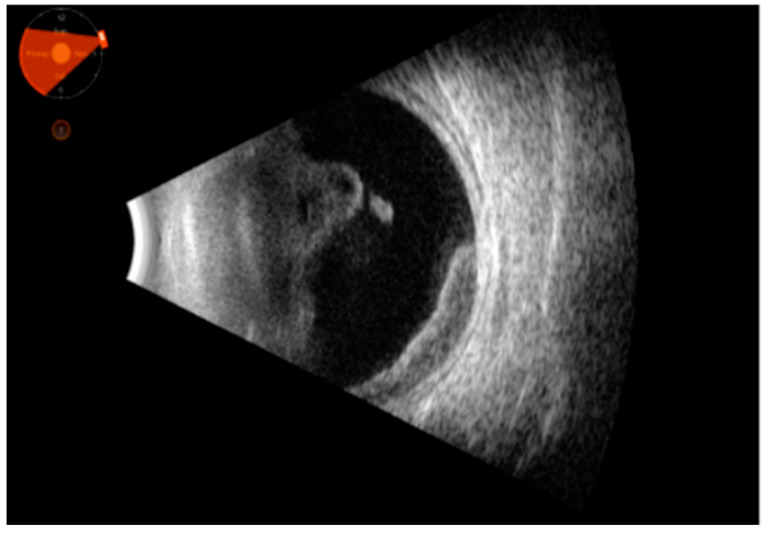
Ultrasound picture of abundant vitreous corpuscles organized into clusters in the anterior vitreous, with high cellularity and mobility. Moreover, the picture shows a highly cellular structure with medium–high reflectivity in the temporal sector, at times in continuity with the vitreous (intraocular lymphoma).
